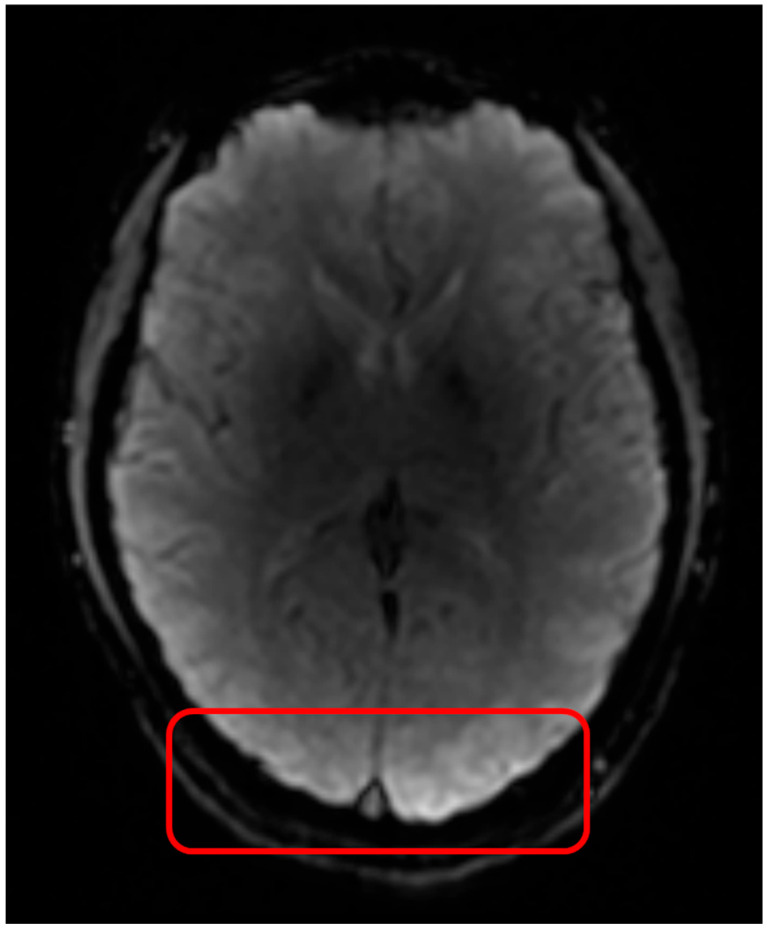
B_1_ field inhomogeneity in DSC MRI appears as spatially varying signal intensities. The red square indicates tissue hyper intensities due to B_1_ field inhomogeneities.
